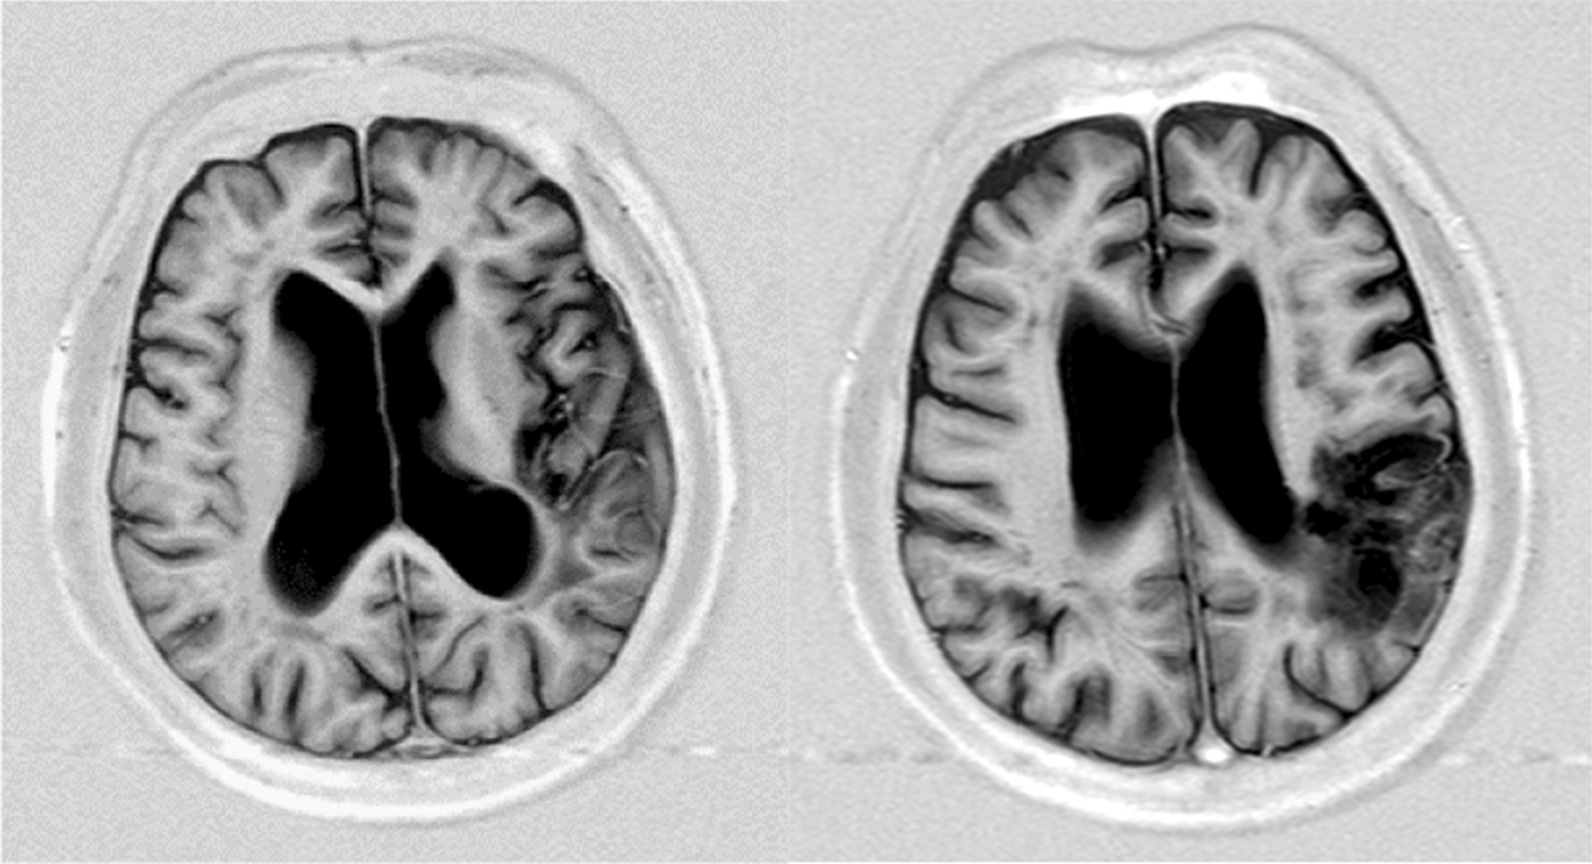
MRI T1 weighted image of the patient’s head. T1-weighted magnetic resonance imaging (MRI) of the head showed low signal regions from the left temporal lobe to supramarginal gyrus and inferior parietal lobule. *MRI*: Magnetic resonance imaging
